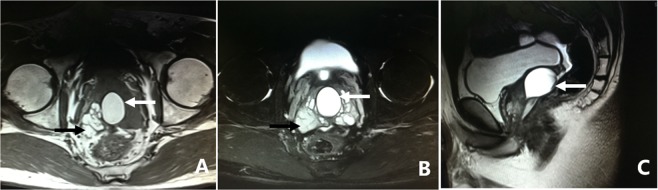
MRI image of MDC. (A) There is a 3.4 × 4.5 cm high-intensity signal cyst in the midline of the prostate, accompanied by dilation and high-intensity signals in the right SV on T1WI. (B) Both the cyst and the bilateral SV present as high-intensity signal on T2WI. (C) The cyst extended beyond the posterosuperior margin of the prostate gland on the sagittal plane. This pattern suggests that the cyst is MDC, accompanied by dilation and an old hemorrhage in the right SV.
